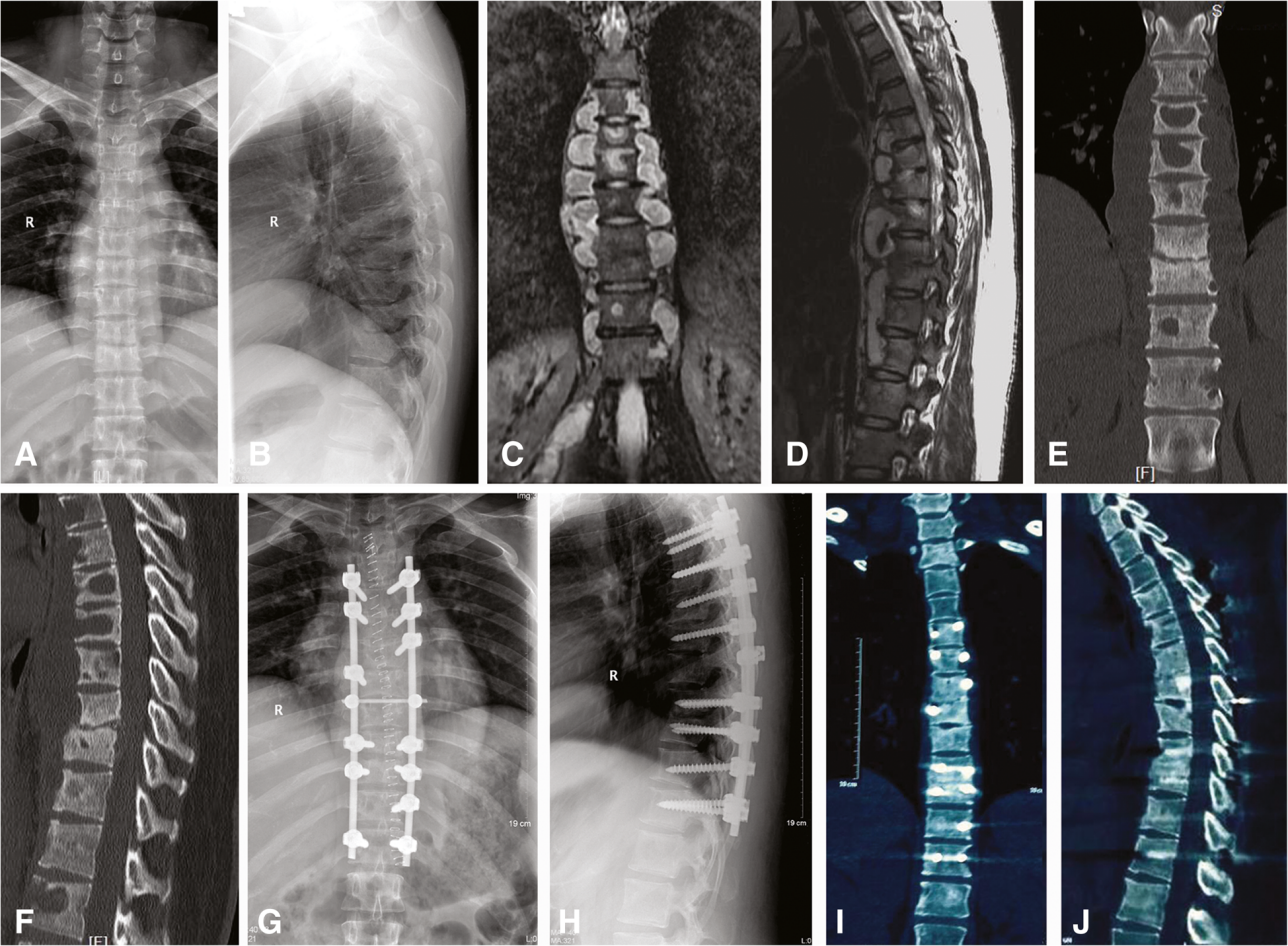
Preoperative radiography (a and b), MRI (c and d), and CT (e and f) of a 24-year-old female patient with tuberculosis at T4–L2 with extensive paravertebral abscesses. Radiography (g, h) and CT (i, j) at the final follow-up showed definitive interbody bone fusion was achieved at T6–7 and T9–10 (e–h). MRI: magnetic resonance image; CT: computed tomography scan (i–j)
